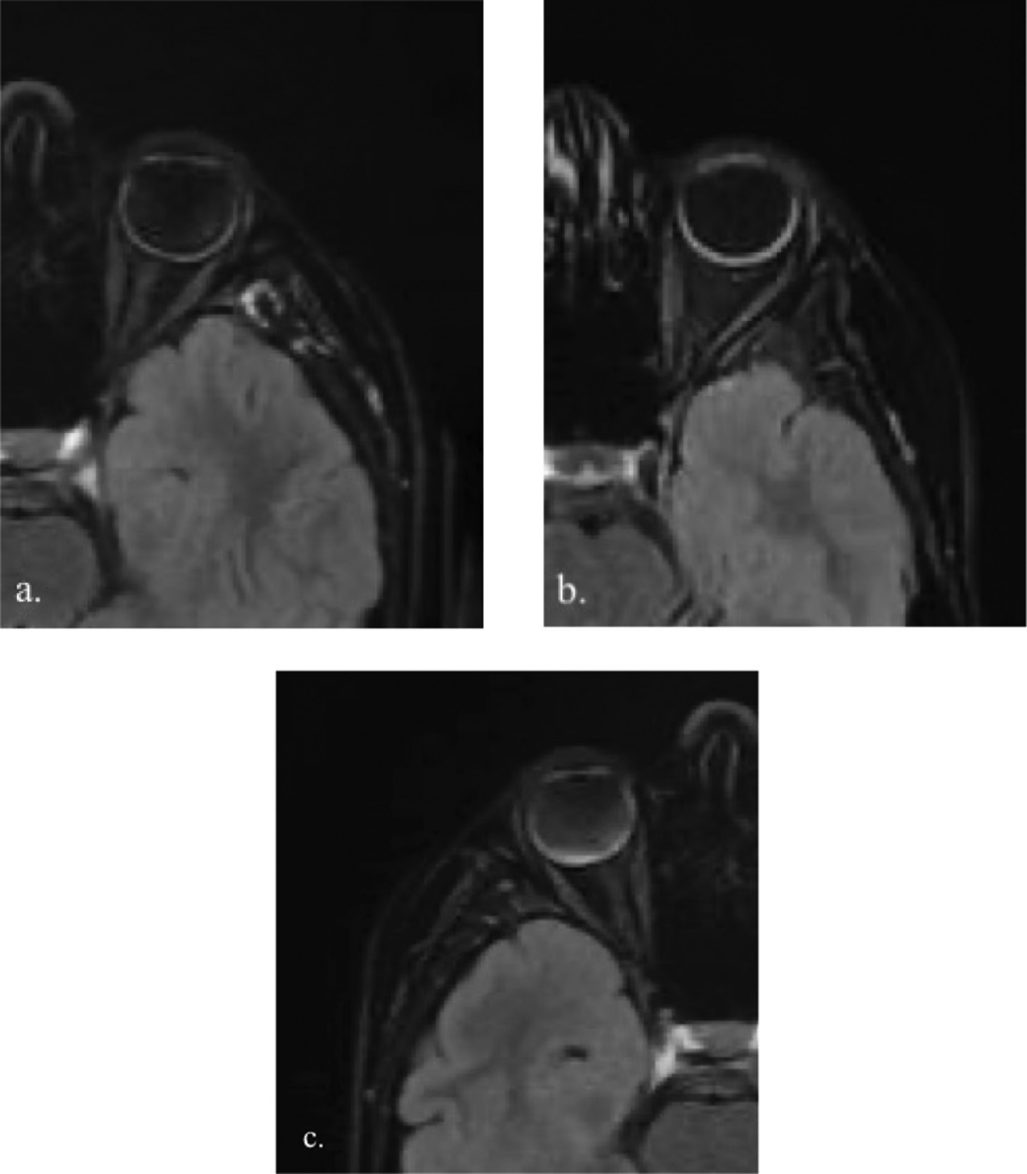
Semi-quantitative assessment of uveitis using CE-FLAIR-FS imaging: mild (a), moderate (b), and marked (c) uveal tract enhancement.
